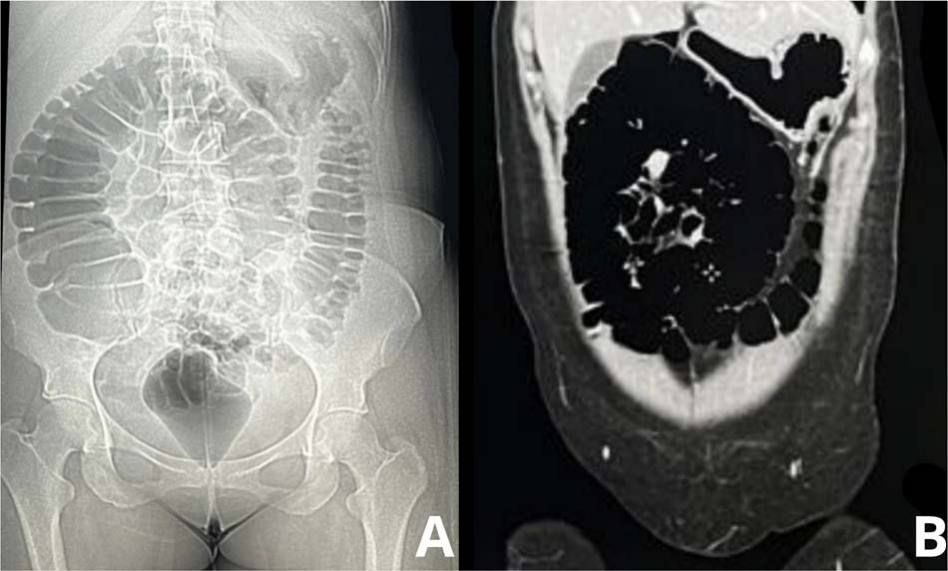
Imaging studies demonstrating ‘Stack of Coins’ sign in small bowel obstruction. A: Plain abdominal radiograph, the abdominal X-ray shows dilated loops of small bowel with visible, closely packed valvulae conniventes, forming the characteristic ‘stack of coins’ appearance. B: Axial CT scan of the abdomen, this contrast-enhanced abdominal CT scan further confirms the small bowel obstruction, with dilated loops filled with gas and fluid.
